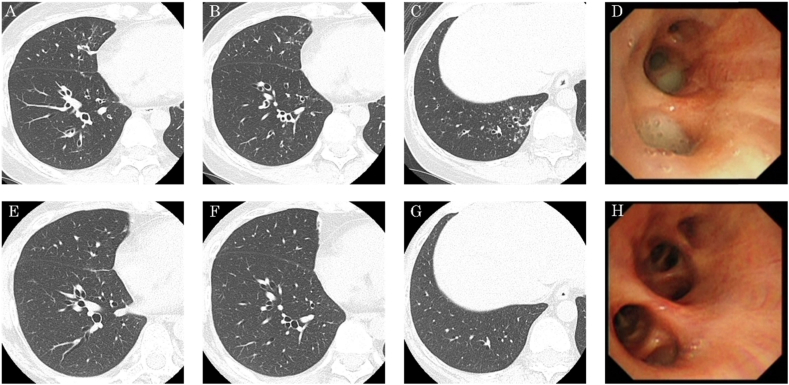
High-resolution computed tomography (HRCT) and bronchial thermoplasty (BT) findings; HRCT showed bronchial wall thickening and infiltrative shadows at the right inferior lobe before the first BT (A, B, C). Endobronchial inspection during the first BT procedure was significant for a large amount of yellow secretion in the right inferior lobe (D). HRCT performed after three BT sessions showed a decrease in bronchial wall thickness and disappearance of infiltrative shadows in the right inferior lobe (E, F, G). Endobronchial inspection during the third BT procedure did not show purulent sputum in the right inferior lobe (H). . (For interpretation of the references to colour in this figure legend, the reader is referred to the Web version of this article.)
